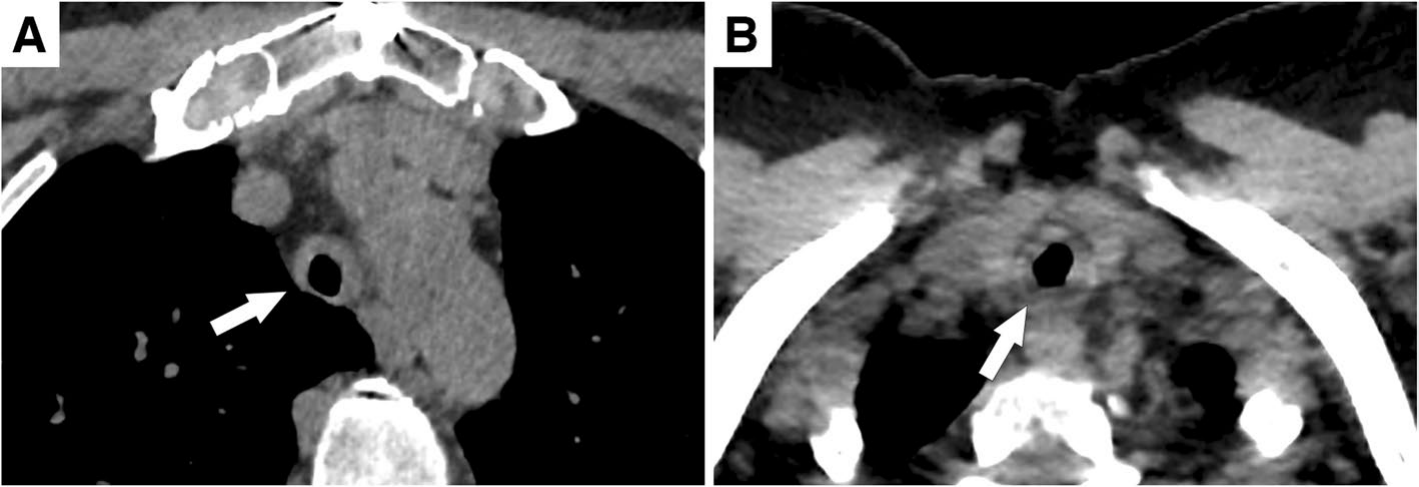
Atypical tracheal wall thickenings. A Unenhanced chest computed tomography (CT) shows tracheal wall thickening sparing the posterior membrane (arrow) in a patient with granulomatosis with polyangiitis. B Unenhanced chest CT showing circumferential tracheal wall thickening (arrow) in a patient with relapsing polychondritis
